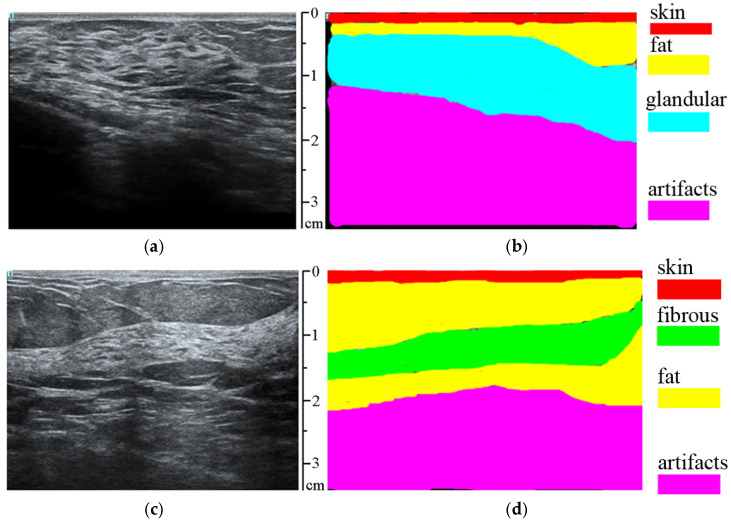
(a,c) Original ultrasound images; (b,d) ultrasound images with marked tissues on them (skin, fat, fibrous tissue, glandular tissue, and artifacts).
